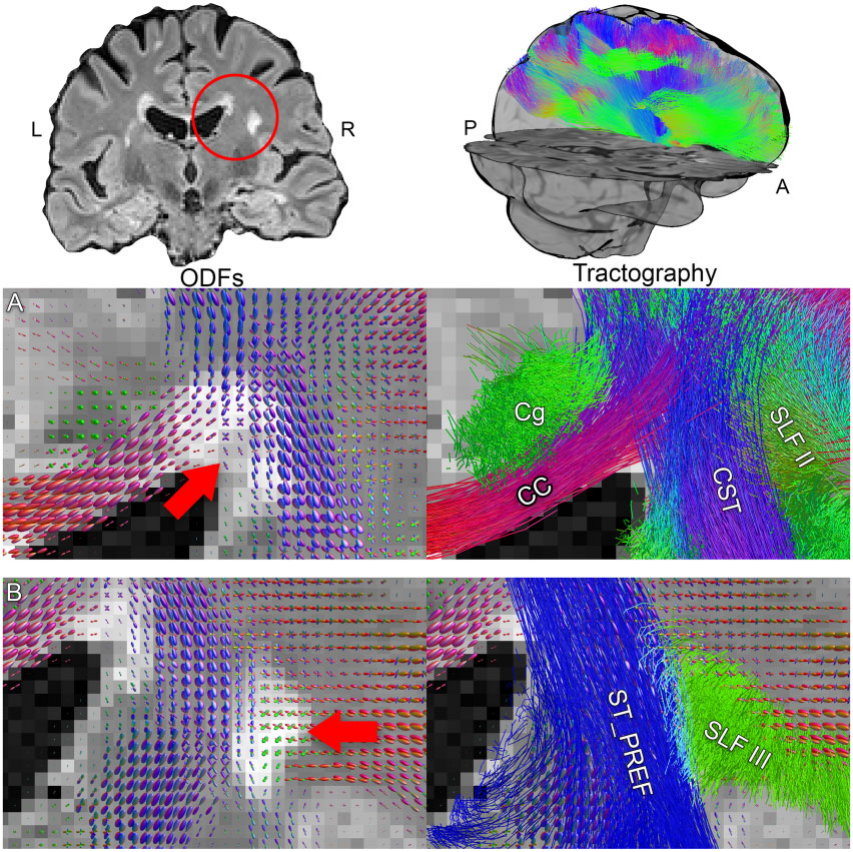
Tractography results in a single participant. Top row: Coronal view of the FLAIR image with lesions highlighted (red circle). On the right, 3D oblique view of the corona radiata and the superior longitudinal fasciculi in the vicinity of the lesion. (A) Fibre orientation distribution functions (fODFs) of the centrum semiovale (red arrow) shows continuity inside and around the lesion, suggesting preserved structural organization.^67^ On the right, multiple major pathways intersect and traverse the lesion (CST, corticospinal tract; Cg, Cingulum; CC, corpus callosum; SLF, superior longitudinal fasciculus). (B) A lesion adjacent to the striato-prefrontal connection (ST_PREF) shows preserved fibre orientations (red arrow) allowing reconstruction of the SLF-III pathway.
